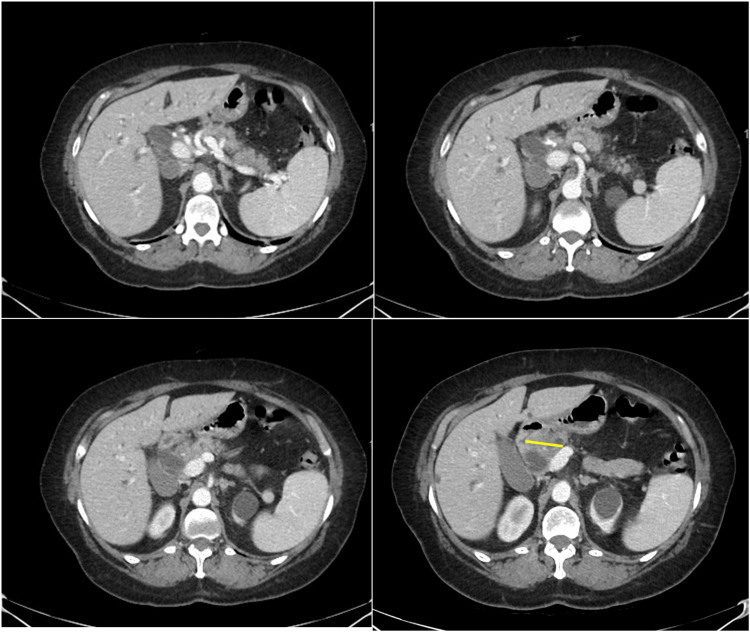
CT imaging of the pancreatic tumour at diagnosis. The yellow bar indicates the longest tumour diameter CT: computed tomography
